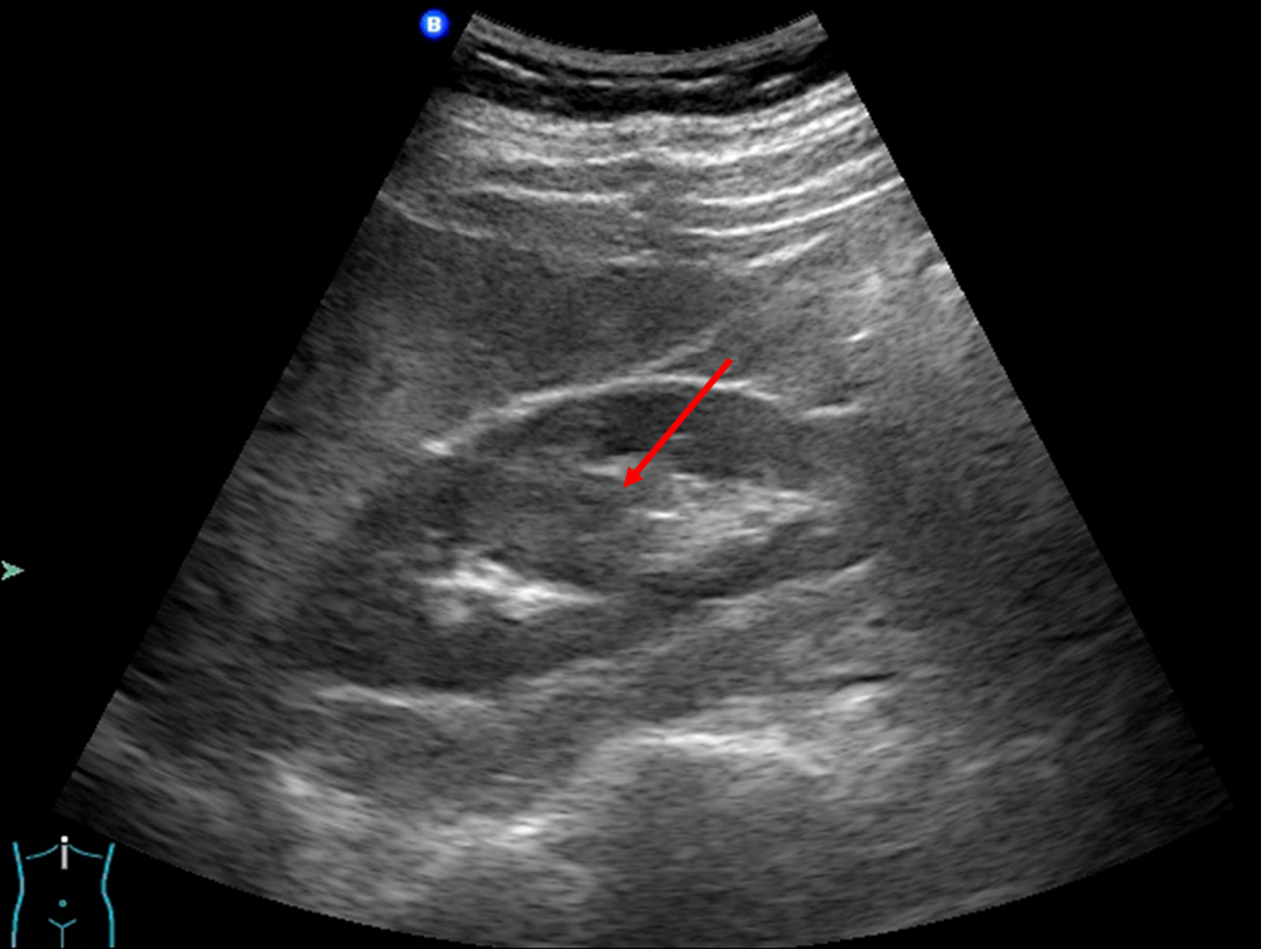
Ultrasound showed a patchy hypoechoic area (red arrow) within the right renal sinus, with slightly irregular shape, clear boundary, the similar echo of renal cortex, and enhanced echo in the rear.
